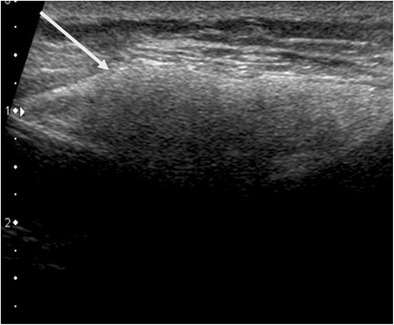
Siliconoma. Periprosthetic silicone (*arrow* showing a hyperechoic mass with attenuation of the ultrasound beam)
